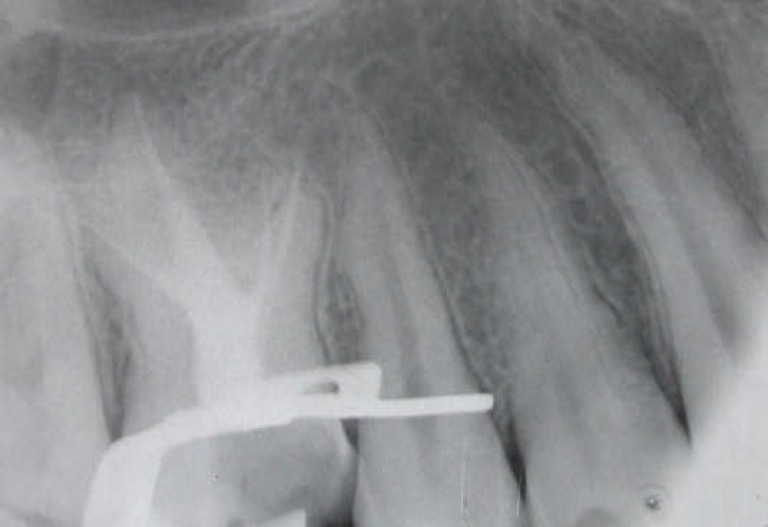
Periapical radiographic images immediately after root canals obturation
